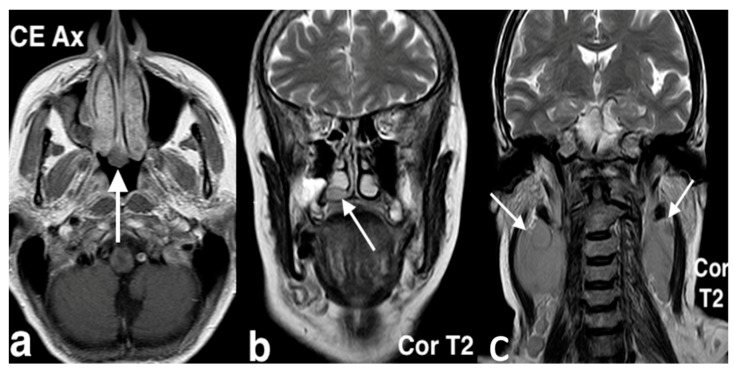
Rhabdomyosarcoma in a 65-year-old woman with nasal rhinorrhea and epistaxis. MRI: the axial T1 after intravenous Gd image (a) shows a nodular tumor (arrow) with low vascularity, attached and eroding the third posterior part of the nasal septum; on the coronal T2 image (b), the mass (arrow) with intermediate intensity is located in the right nasal fossa floor, between the inferior turbinate and nasal floor; the coronal T2 image (c) shows bilaterally metastatic lymph nodes in II-III levels (arrows).
